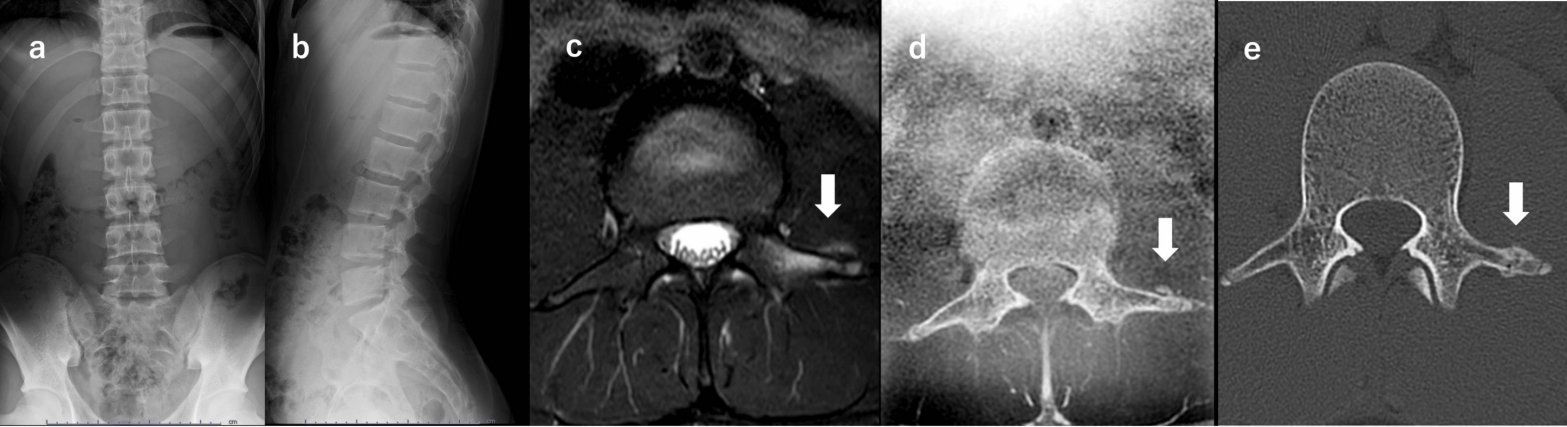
Sequential imaging findings of an L3 transverse process stress fracture in Case 1 (a, b) Plain radiographs showing no abnormalities, (c) Axial Short Tau Inversion Recovery (STIR) MRI demonstrating high signal intensity at the left L3 transverse process and adjacent soft tissue (white arrow), (d) MR bone imaging revealing a fracture line and callus-like change (white arrow), (e) Axial CT image at four weeks confirming bone union (White arrow).
